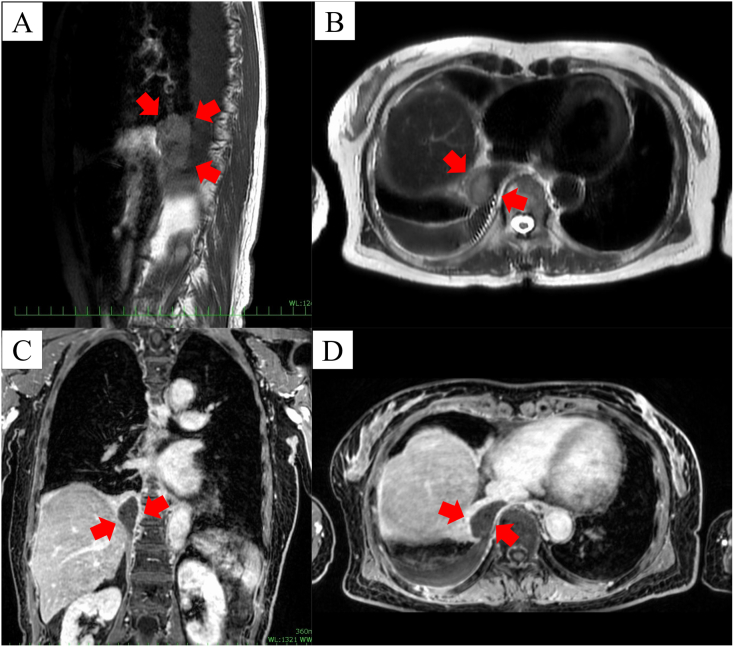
Magnetic resonance imaging findings. T1-weighted sagittal imaging shows a mass with predominantly low signal intensity (red arrows). (C) T2-weighted imaging shows a well-marginated oval mass (red arrows) with mild pleural effusion. (B) Contrast-enhanced T2 weighted coronal image shows a hypointense mass (red arrows) in the inferior medial right pleural space. (D) Contrast-enhanced T2 weighted axial image showing a homogeneous low signal in the mass (red arrows). (For interpretation of the references to colour in this figure legend, the reader is referred to the web version of this article.)
